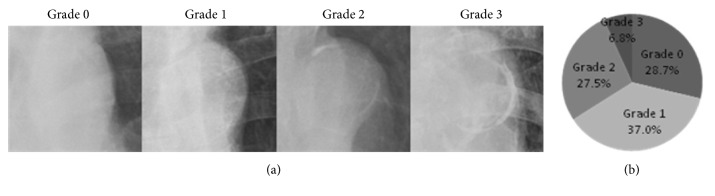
The aortic arch calcification (AAC) extent in four-point scale and distribution of AAC grades on a chest X -ray. (a) The extent of AAC on a chest X-ray was divided into four grades: grade 0, no visible calcification (panel A); grade 1, small spots of calcification or a single thin area of calcification of the aortic knob (panel B); grade 2, one or more areas of thick calcification (panel C); grade 3, circular calcification of the aortic knob (panel D). (b) Distribution of AAC grades on chest X-ray in all subjects.
